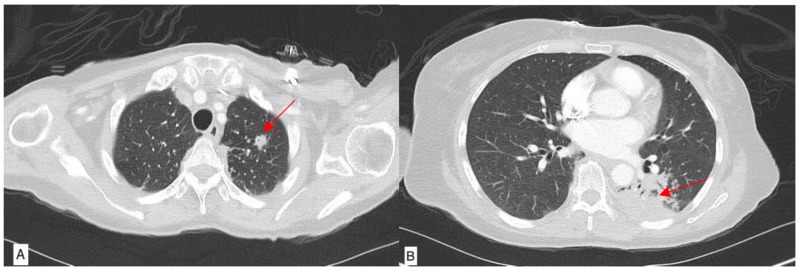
Computed tomography (CT) of the chest showing (3A) pulmonary nodule at the left lung apex (arrow), (3B) dense area of consolidation in the left lower lobe (arrow)
